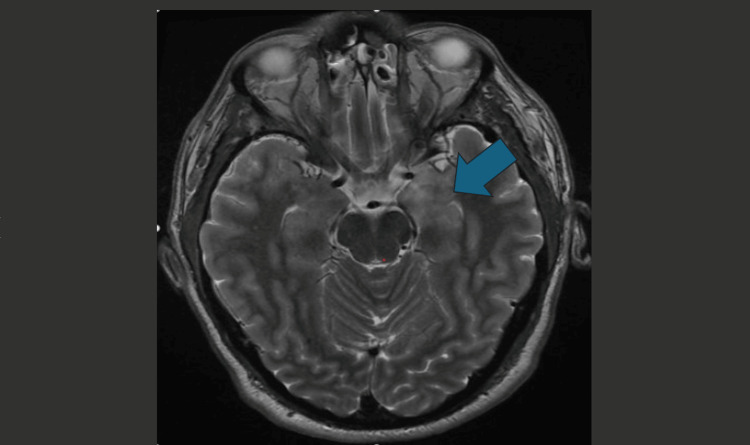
MRI head with contrast showing abnormal signal involving the left medial temporal lobe, eliciting bright T2 and low T1 signal with mild contrast uptake.
